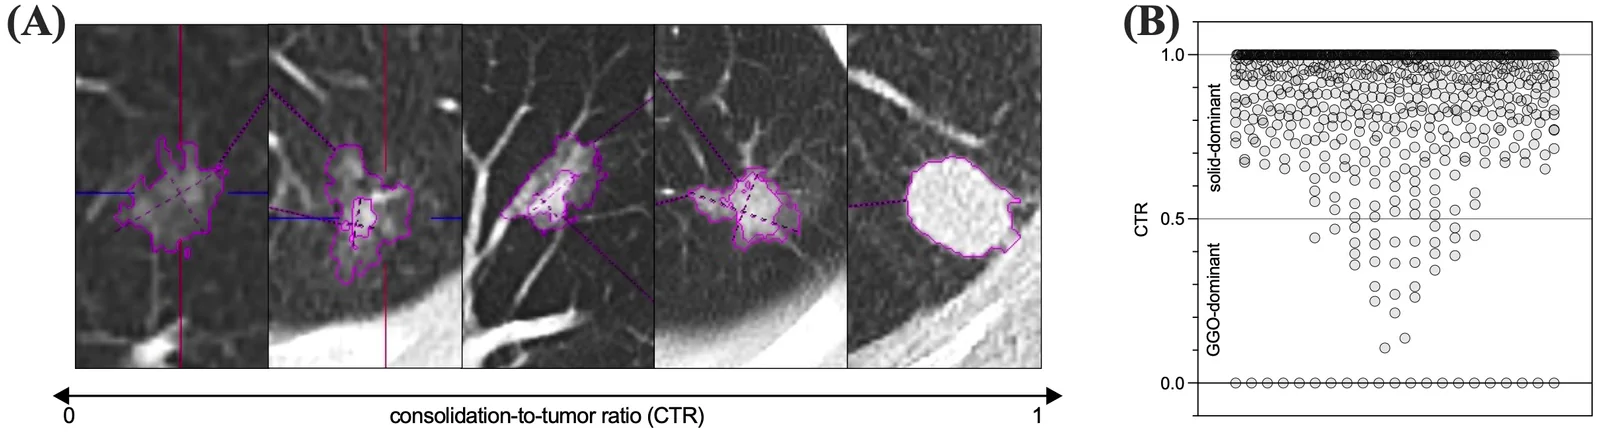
Assessment of consolidation-to-tumor ratio (CTR) on preoperative computed tomography images. (A) Representative preoperative chest CT images with measurement of solid and GGO-components in syngo.via. (B) Distribution of CTR in the study population. Dots represent individual patients. Patients were classified as GGO-dominant (CTR ≤ 0.5) or solid-dominant (CTR > 0.5).
